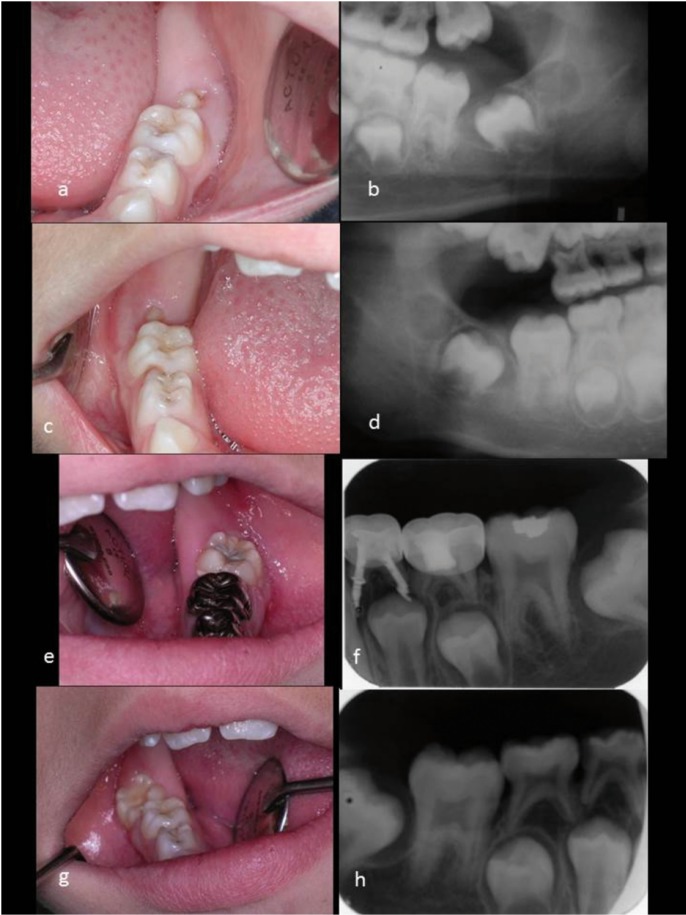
A to D: Follow up, three months after the first surgical intervention. a) Intraoral image section 30. b) Radiograph of section 30. c) Intraoral image section 40. d) Radiograph of section 40. E to H: Follow up, two years after the first surgical intervention. e) Intraoral image section 30. f) Radiograph of section 30. g) Intraoral image section 40. h) Radiograph of section 40.
